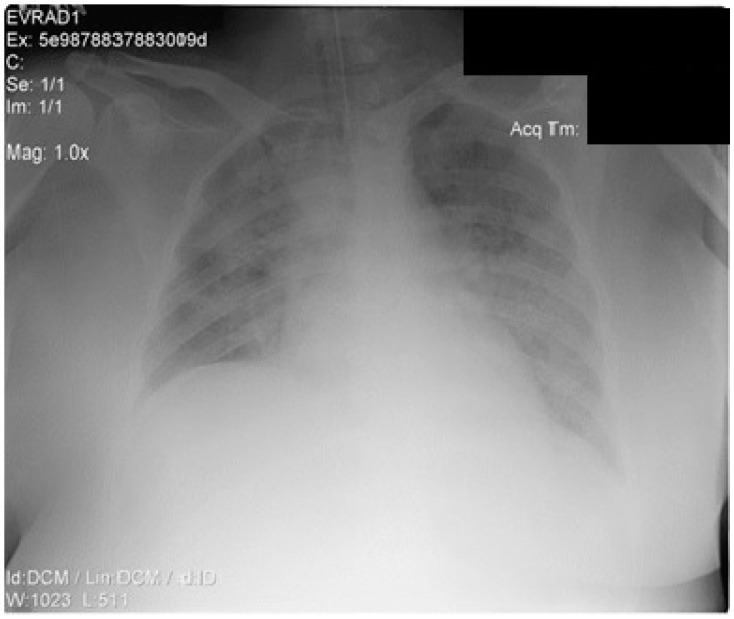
Thorax X-ray after intubation (anteroposterior view).
